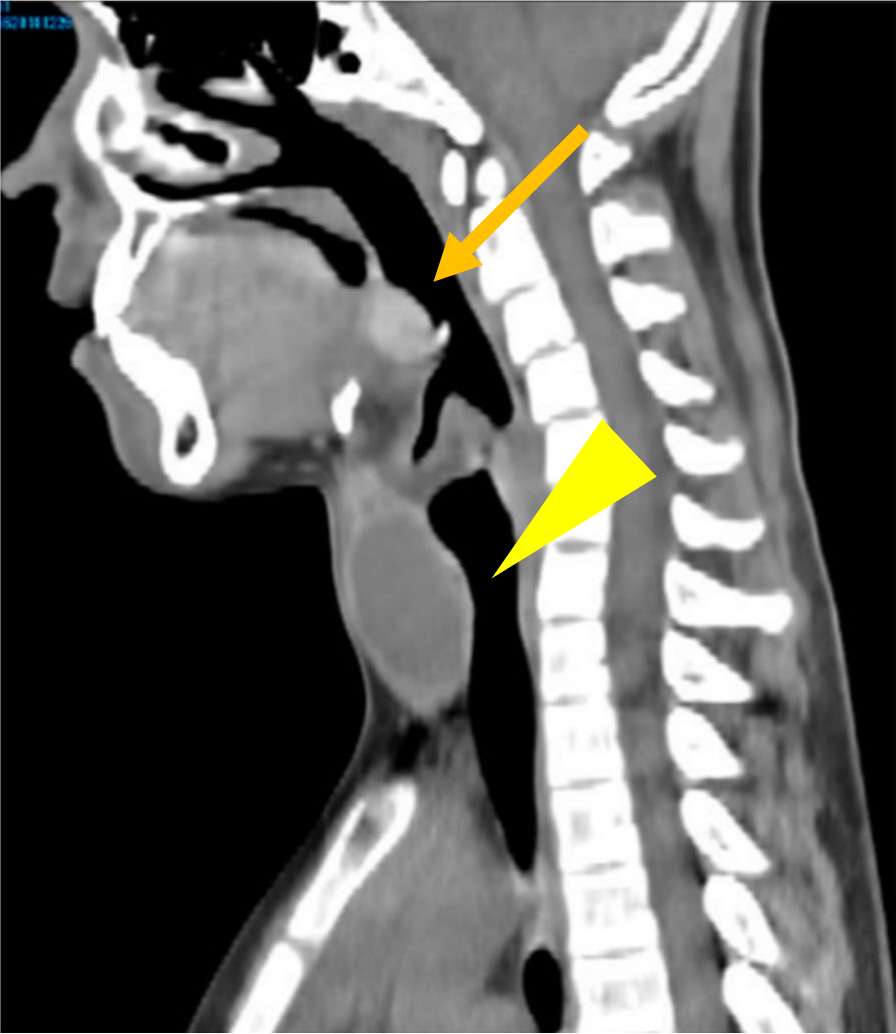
Preoperative CT shows a mass in the sublingual region (arrow) and the development of the pretracheal region below the hyoid (arrowhead)
